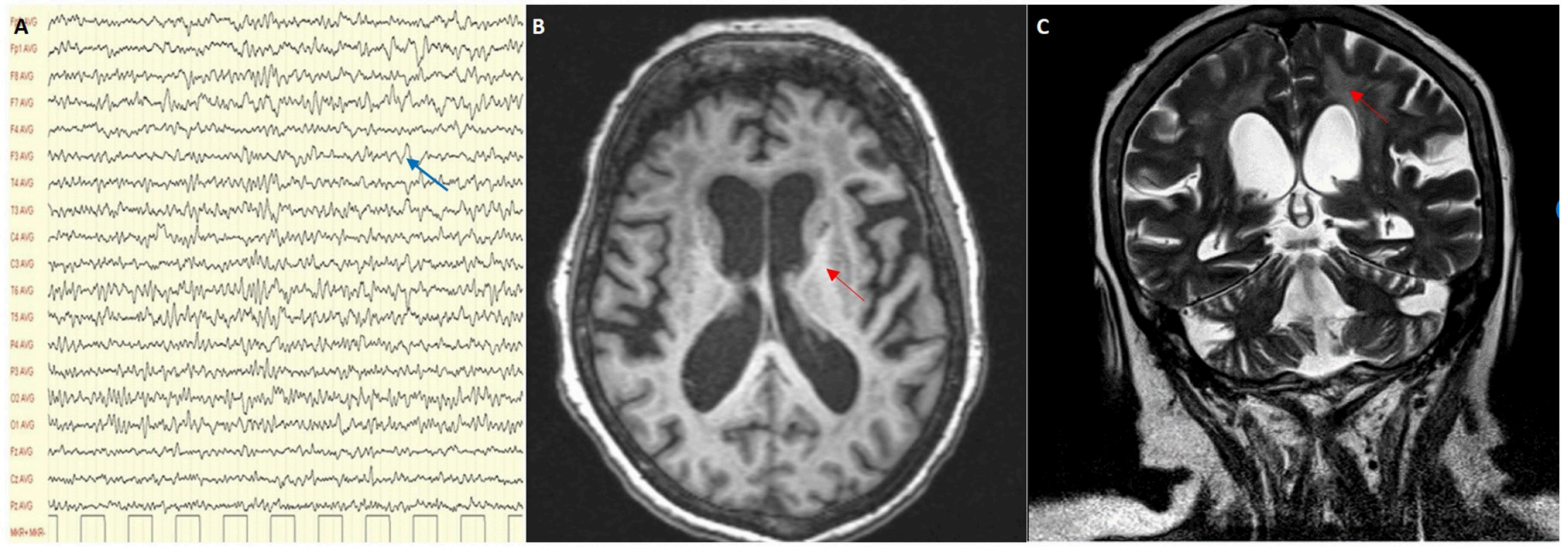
(A) EEG showing a slow alpha with theta and delta activity (blue arrow); (B,C) MRI scan showing cortical and subcortical atrophy with confluent areas of white matter hyperintensity (red arrows) in T1 and T2-weighted images.
